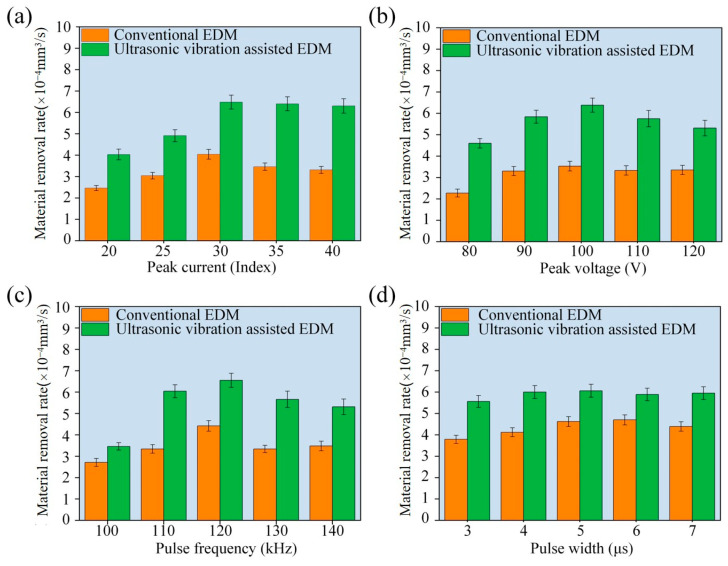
Effect of electrical parameters on MRR in conventional EDM and ultrasonic vibration-assisted EDM. (a) Peak current; (b) peak voltage; (c) pulse frequency; (d) pulse width.
